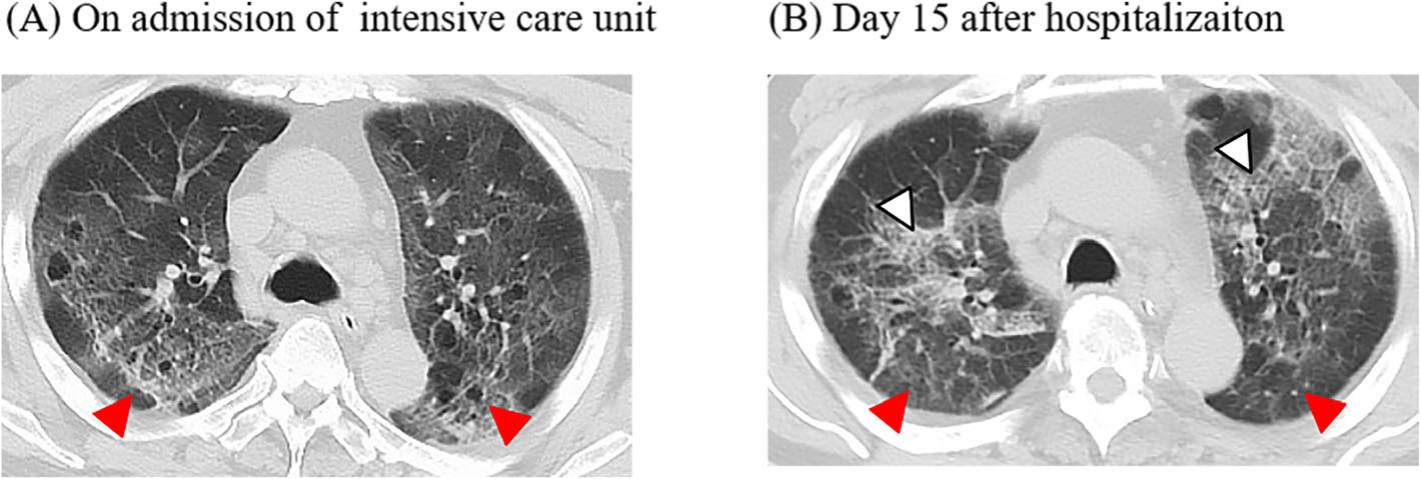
Computed tomography (CT) scan of the chest on admission to the intensive care unit (a) and on Day 15 after admission (b)
